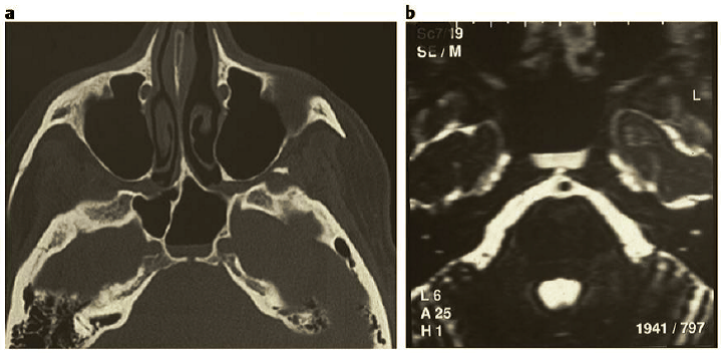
Female patient with condition after pneumococci meningitis and bony defect of the posterior wall of the sphenoid sinus (CT scan) as well as liquor passage into the sphenoid sinus (MRI). a) axial CT scan. b) MRI (CISS sequence).
